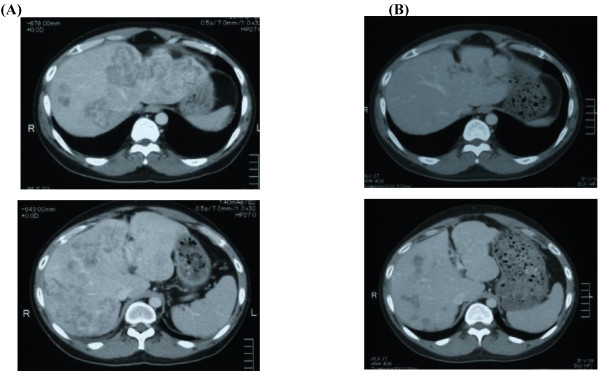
Reduction of tumor volume in the liver after the cetuximab and irinotecan treatment. (a) Computed tomography before and (b) after the combination treatment with cetuximab and irinotecan.
